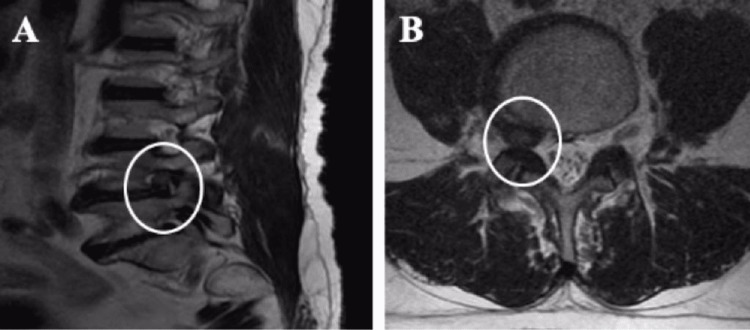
Sagittal and axial T2 image of right L4-L5 FLLDH on preoperative lumbar MRI FLLDH: far lateral lumbar disc herniation
